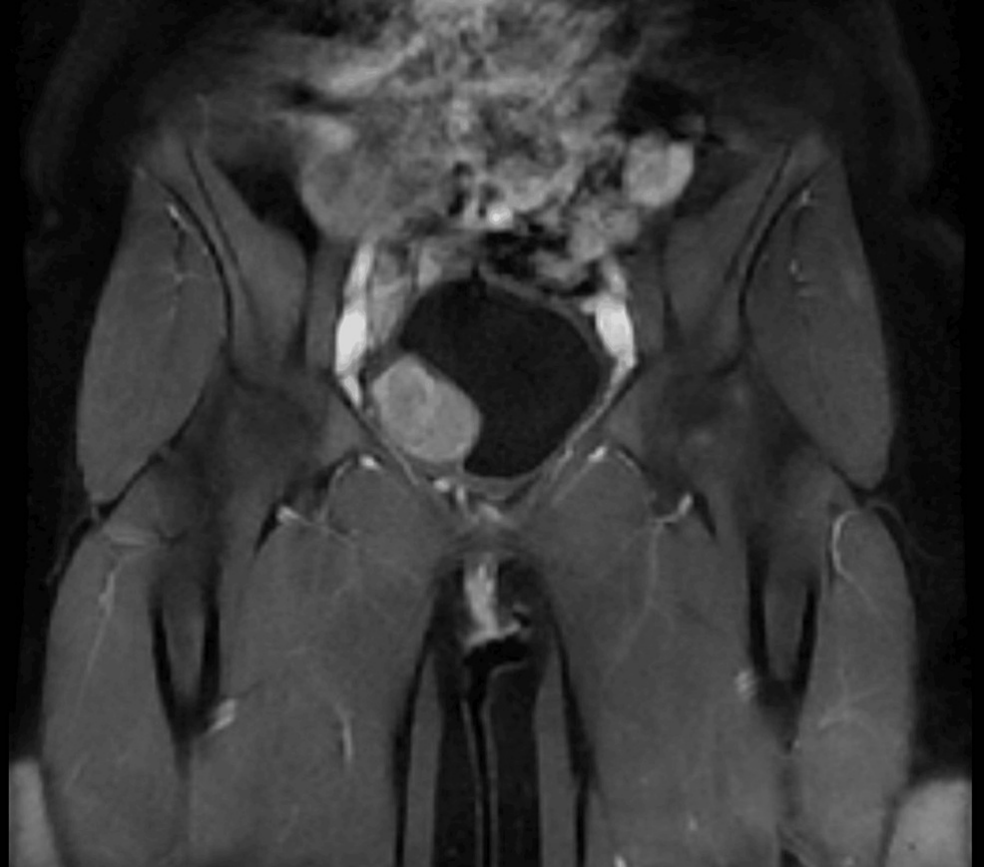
Pre-operative contrast-enhanced pelvic MRI T1 coronal
